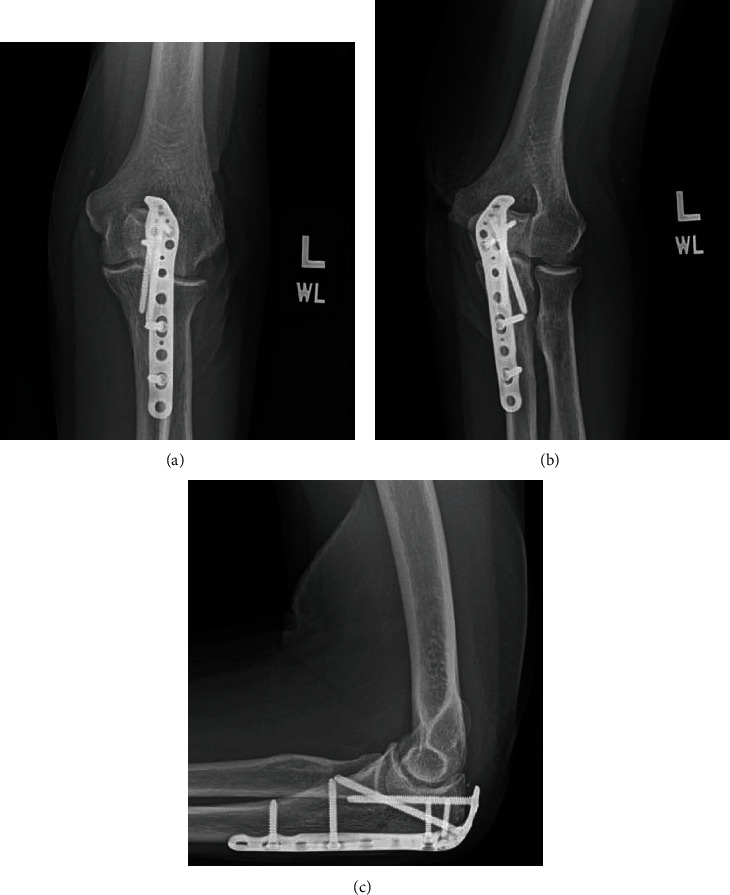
AP (a), oblique (b), and lateral (c) radiographs of the patient's left olecranon at the 3-month follow-up visit after operative fixation.
